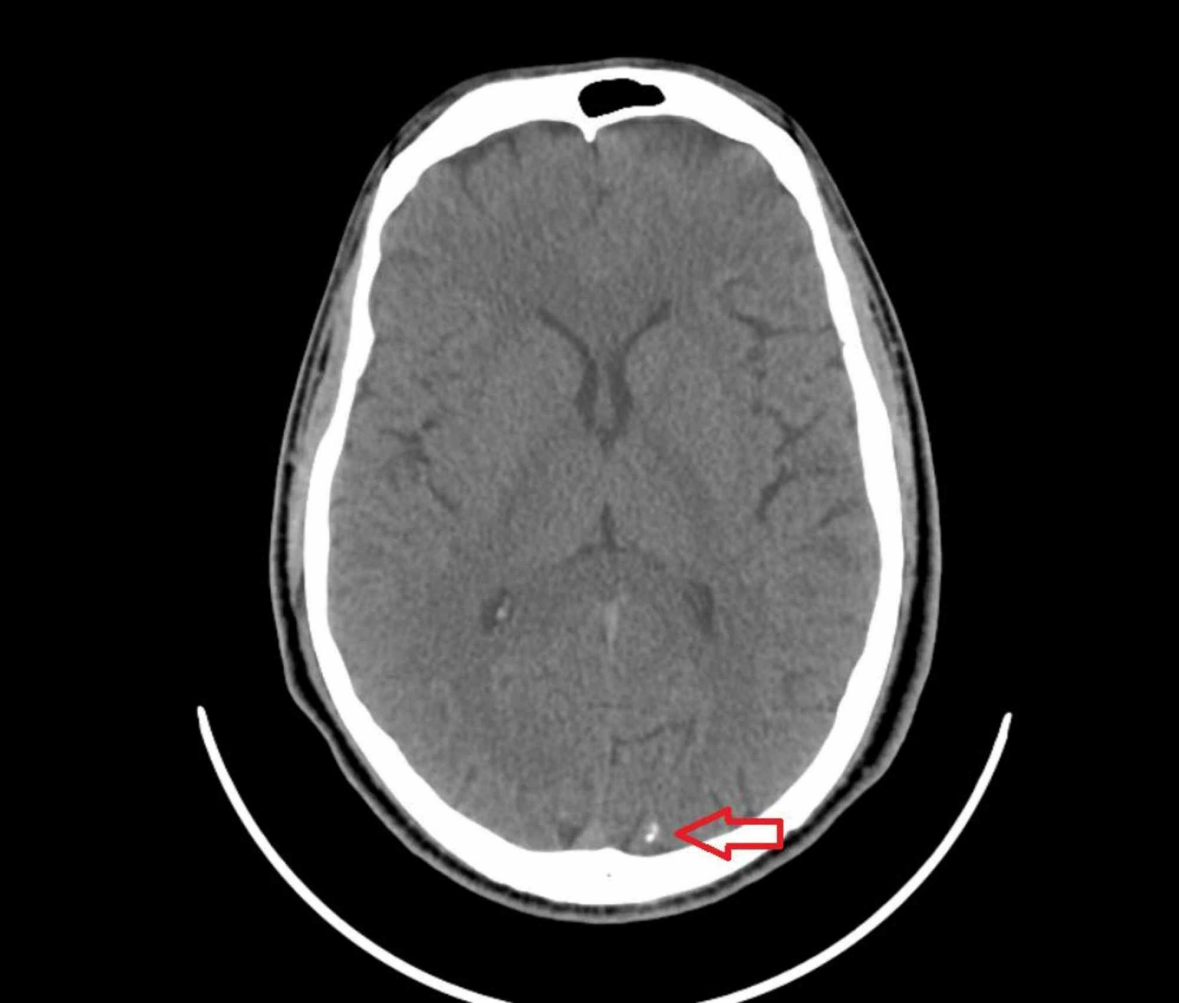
Computed tomography of the head showing a small focus of intraparenchymal calcification in the left occipital lobe
